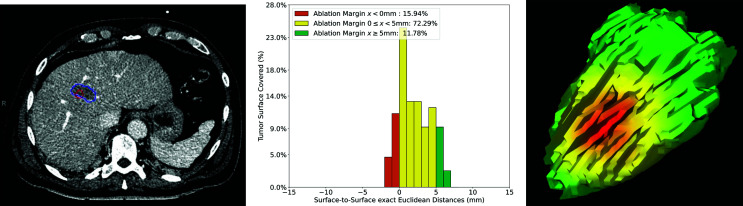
Volumetric assessment of quantitative ablation margins (QAM) in a patient treated with SMWA for a CRLM in liver segment VIII. Left: Computed tomogram image with segmented tumor (red) and ablation (blue) volumes. Center: Relative frequencies of ablation margin substrata with respect to percentage of tumor surface covered. Right: 3D representation of the ablation margin projected onto the ablation surface, showing the area of negative margins in red.
